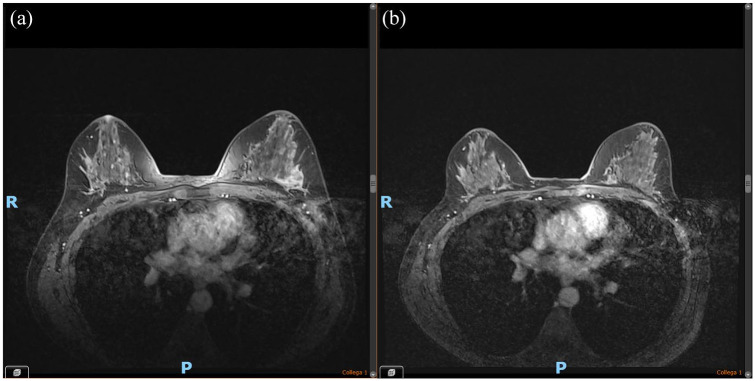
Breast MRI of Twin B. (a) Baseline. (b) Post-neoadjuvant therapy.
